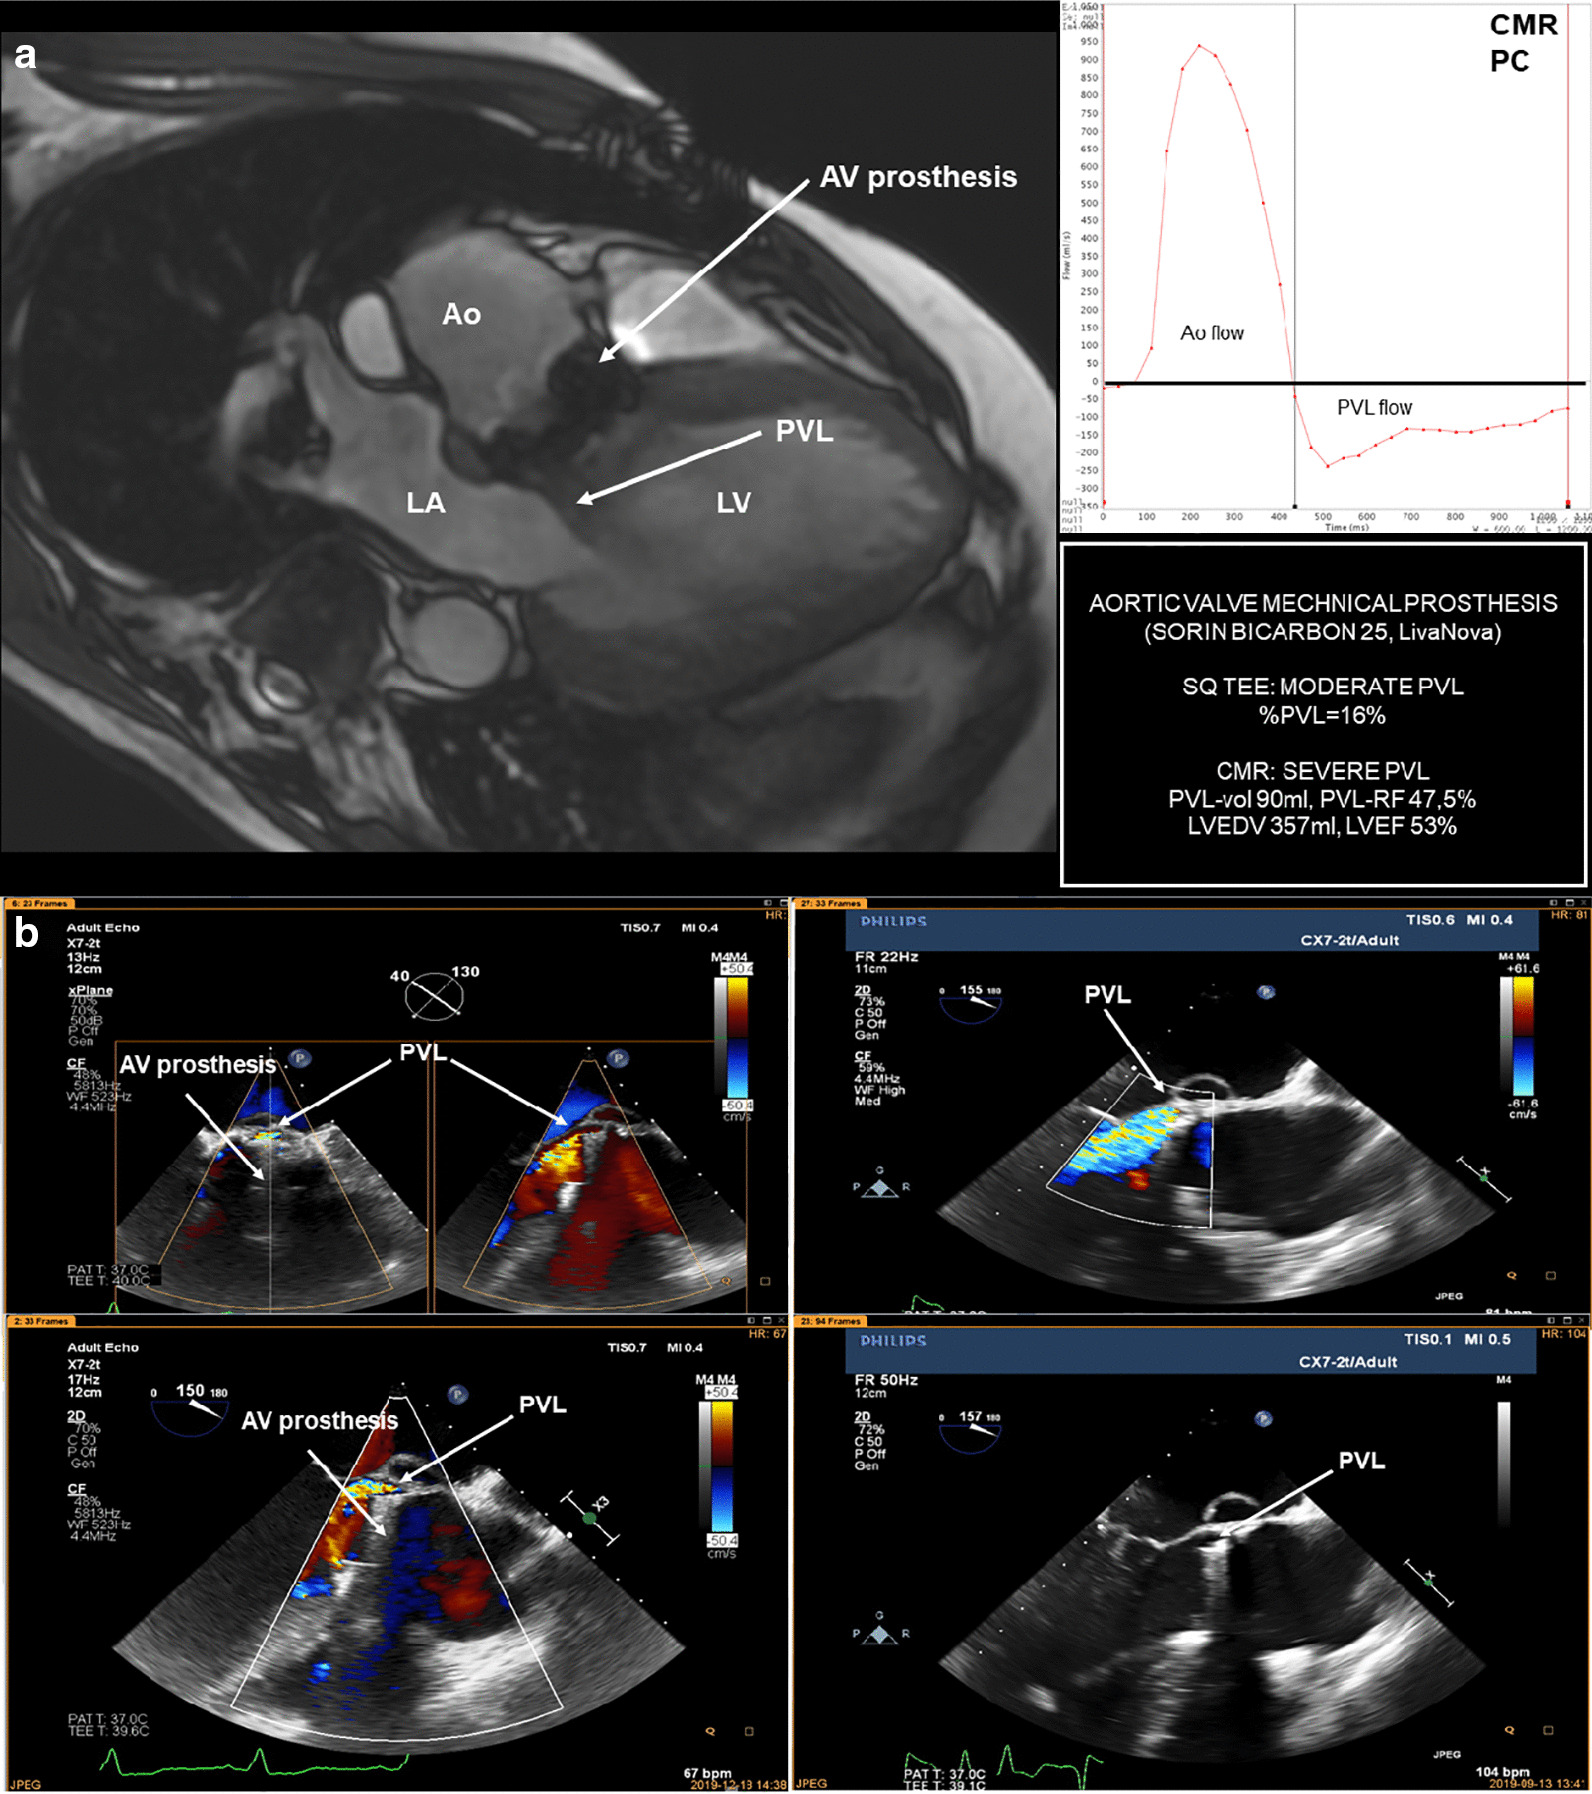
The case of the study patient with a paravalvular leak related to the aortic valve (AV) mechanical prothesis in cardiovascular magnetic resonance (a) and transesophageal echocardiography (b). *Ao* aorta; *AV* aortic valve; *EDV* end-diastolic volume; *EF* ejection fraction; *LA* left atrium; *LV* left ventricle; *PVL* paravalvular leak; *%PVL* the percentage of the PVL jet circumference related to the prosthetic valve circumference; *PVL-vol* paravalvular leak volume; *PVL-RF* paravalvular leak regurgitation fraction; *SQ* semi-quantitative
